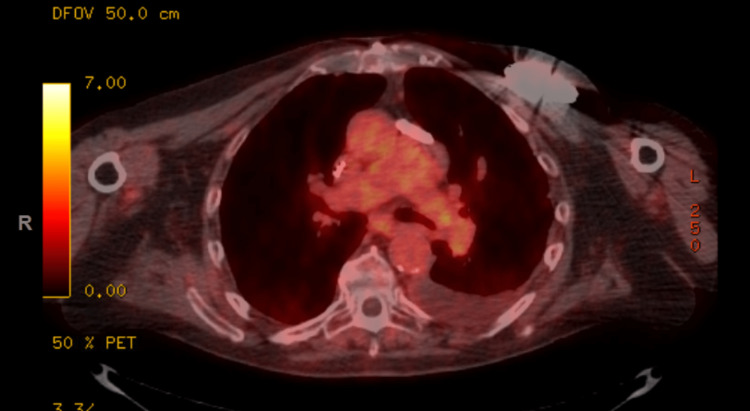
Interval improvement in FDG-avid mediastinal lymphadenopathy after two months of bexarotene treatment FDG: 18F-fluorodeoxyglucose
